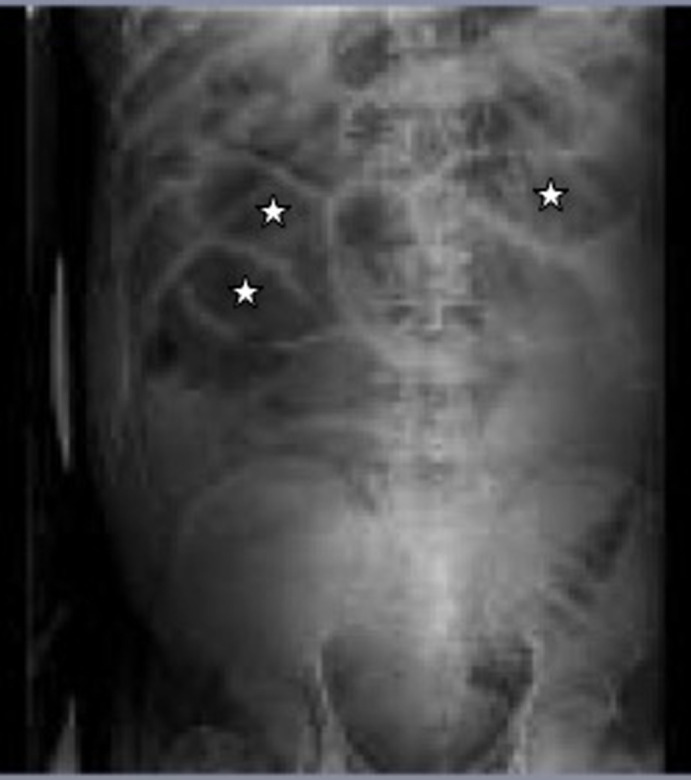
radiographie de l'abdomen sans préparation du 2^e^ patient montrant des aérocolies (astérisque) sans signe de perforation
